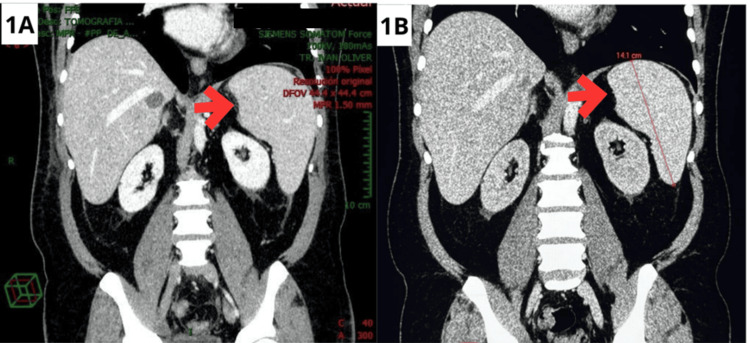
Abdominal contrast-enhanced computed tomography (CECT) coronal multiplanar reconstruction (MPR) obtained during the portal venous phase (A) Coronal image obtained at the initial evaluation demonstrating severe splenomegaly, with a calculated splenic volume of 605.0 cc (arrow). (B) Follow-up coronal image obtained six months later demonstrating progression of splenomegaly, with an increased splenic volume of 631.6 cc (arrow). The images highlight the significant mass effect exerted on adjacent abdominal structures.
